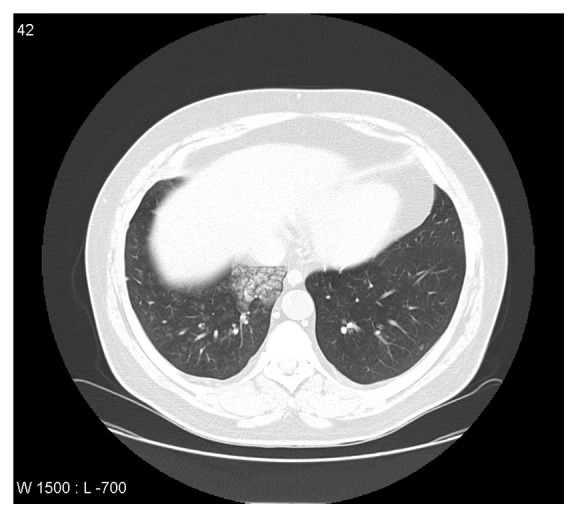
Chest CT scan showing alveoral hemorrhage and consolidation in the right middle lobe.
